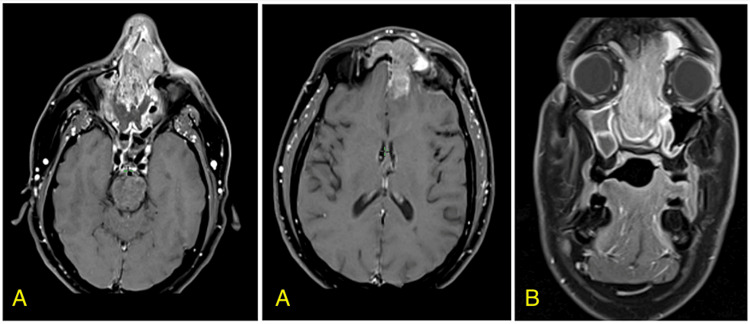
Pre-treatment axial (A) and coronal (B) T1 post-contrast MR images
